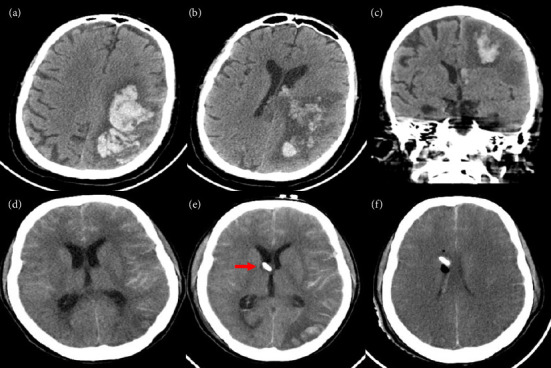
CT brain images (a–c) of a 56-year-old man presented with electrical storm that required VA-ECMO support and developed ICH after 6 days of ECMO support. CT brain images (d–f) of a 28-year-old female who developed subarachnoid hemorrhage (SAH) after 4 days of VA-ECMO support for cardiogenic shock due to severe pulmonary hypertension. Brain death occurred after SAH despite ventricular drain (red arrow).
